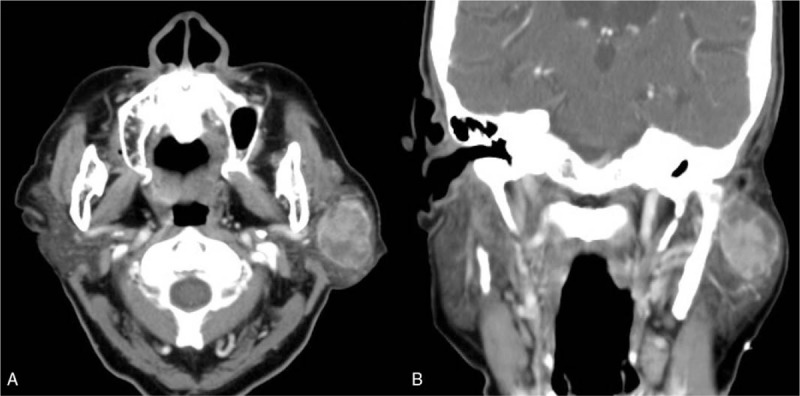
Head and neck CT scan showing a 5.5 × 3.3 cm radiopaque mass lesion with lobulated fluid accumulation crossing over the parotid gland in axial view (A) and coronal view (B). CT = computed tomography.
